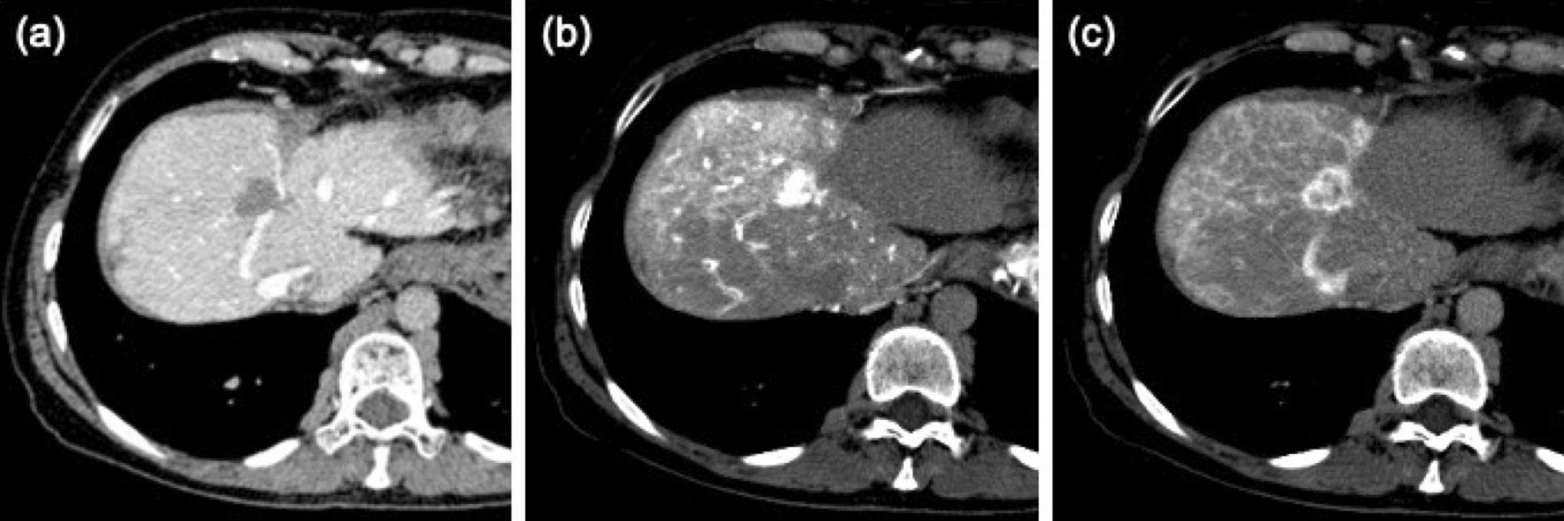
Computed tomography images during angiography at the time of S8 nodule diagnosis in 2007. a Computed tomography (CT) during arterioportography image showing a defect in the portal supply to the tumor. b, c CT during right hepatic arteriography images showing overt enhancement followed by corona-like enhancement, a typical presentation of hepatocellular carcinoma
